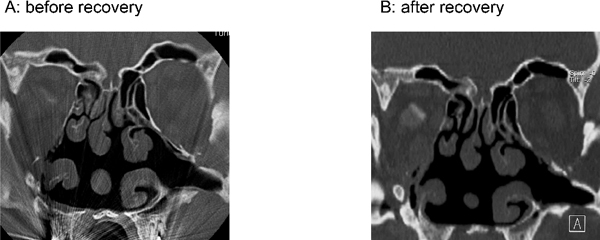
Computed tomography scans before and after recovery of olfactory function. Both scans (before [A, left] and after [B, right] recovery from post-traumatic anosmia) show the same anatomical structures. There was no sign of either conductive olfactory loss due to obstruction of the olfactory cleft, or sinunasal disease.
